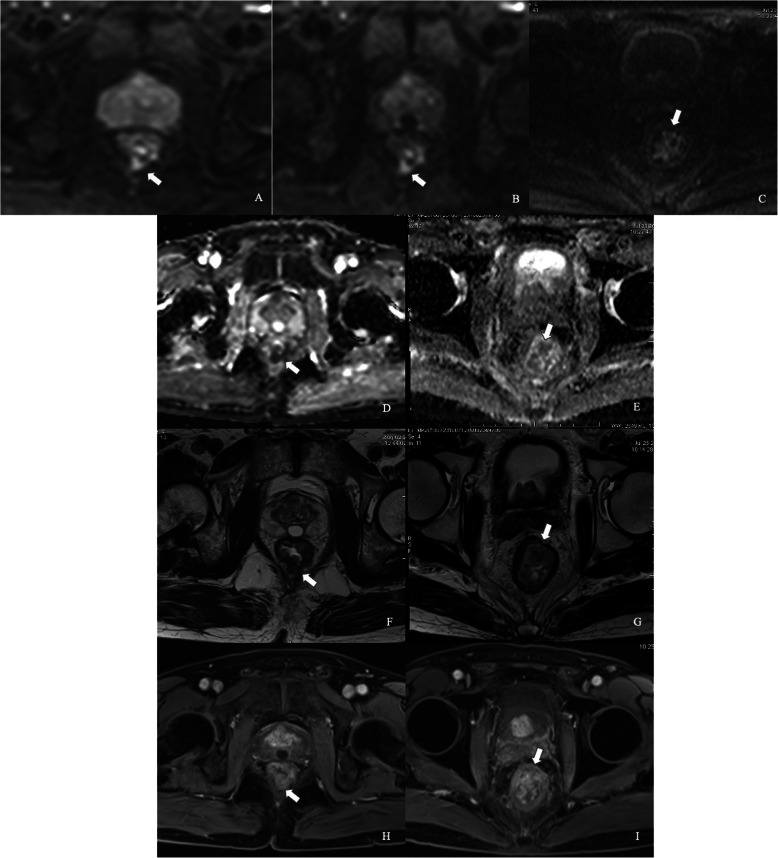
Comparison between iShim- and SS-EPI-DWI in patients after CRT. iShim-DWI of b = 800 s/mm^2^ (a) and b = 1600 s/mm^2^ (b) showed higher SNR and CNR with lower signal noise compared with SS-EPI-DWI of b = 1000 s/mm^2^ (c). ADC map (e) of iShim-DWI showed better image quality compared with ADC map (f) of SS-EPI-DWI. T2W images (f and g) and dynamic T1W images (h and i) showed the same location of lesion (arrows) for iShim- and SS-EPI-DWI cohort, respectively
